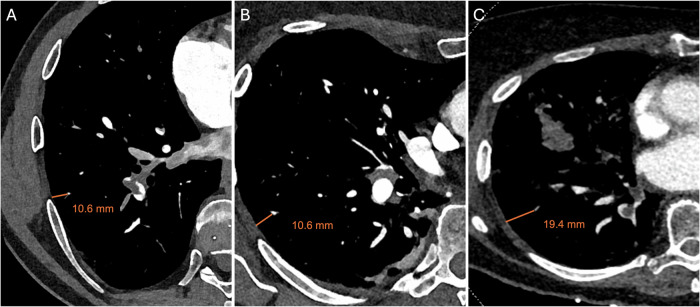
Increased peripheral pulmonary artery visibility with ultra-high-resolution photon-counting CT. UHR-PCD-CTPA at IQ50 (A) and IQ25 (B) vs. EID-CTPA (C). Representative measurements of the minimal distance of the last discernible pulmonary artery branch to the pleura are shown (orange line, in mm). CTPA, CT pulmonary angiography; EID, energy-integrating detector; IQ, image quality index; PCD, photon-counting detector; UHR, ultra-high resolution
